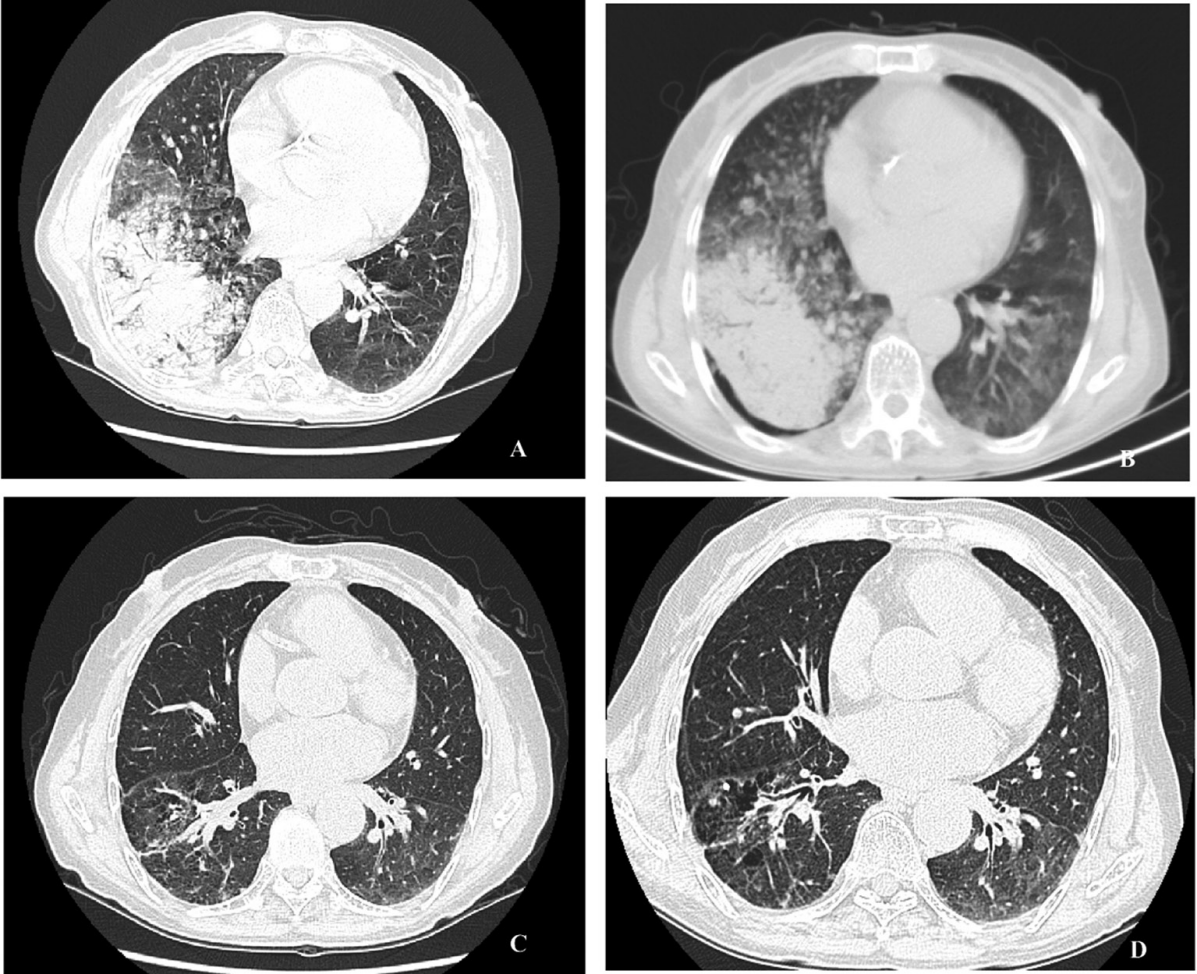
A: Initial chest CT scan, a consolidative mass in RML and RLL with multiple hematogenous metastatic nodules in BUL. B: Chest CT scan before treatment with 250 mg gefitinib, more increased consolidative mass in the right lung field. C: Chest CT scan 6 months after treatment with 250 mg gefitinib, much improved consolidative mass in the right lung and markedly improved hematogenous metastasis in BUL. D: Chest CT scan 13 month after treatment with 250 mg gefitinib, no interval change compared with the previous CT scan.
